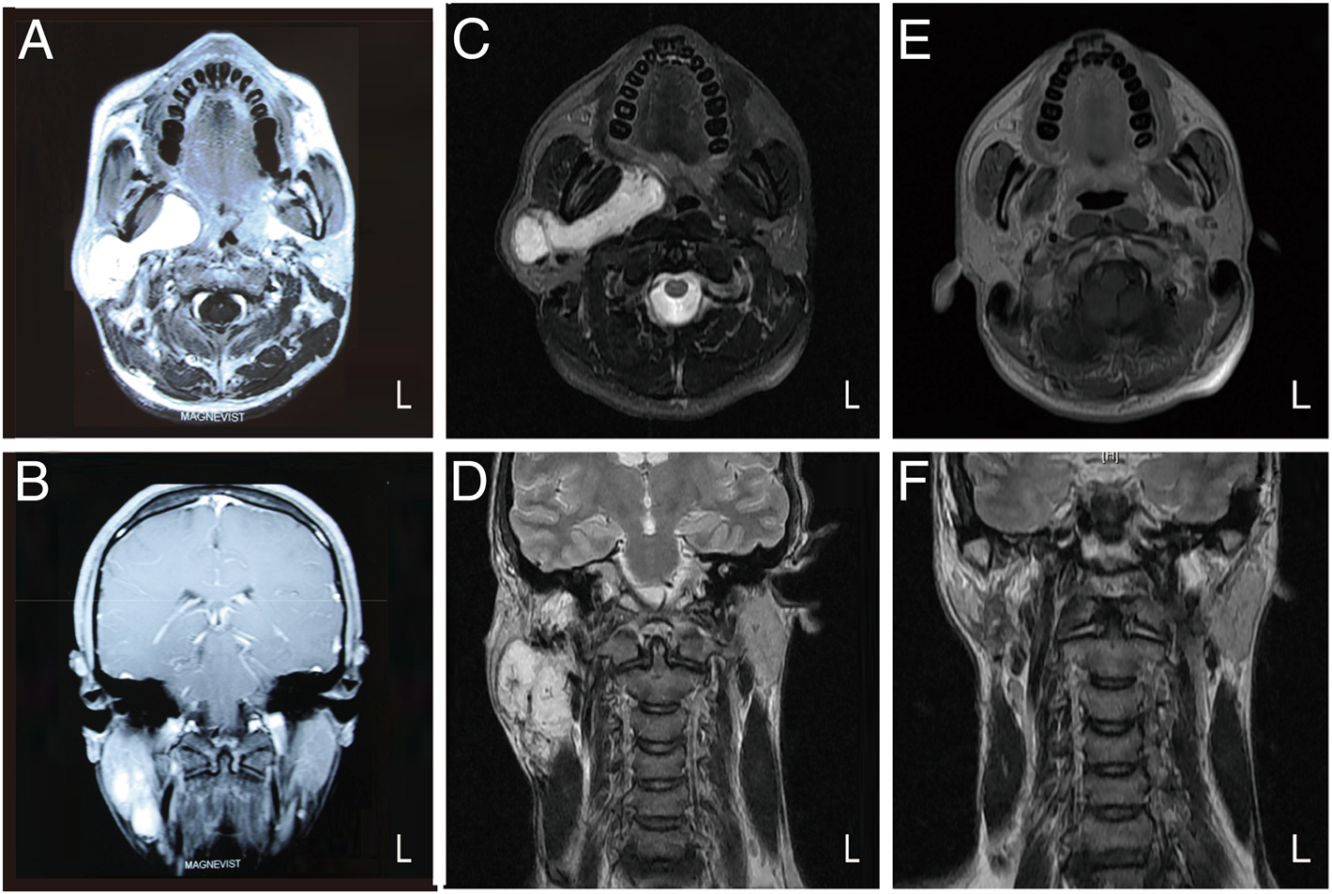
Giant cell angiofibroma. (A) Axial T1-weighted MRI of the tumor prior to sclerotherapy. (B) Coronal T1-weighted MRI of the tumor prior to sclerotherapy. (C) Preoperative axial T2-weighted MRI of the tumor after sclerotherapy. (D) Preoperative coronal T1-weighted MRI of the tumor after sclerotherapy. (E) Postoperative axial T1-weighted MRI at six months after resection showing no signs of recurrence. (F) Postoperative coronal T1-weighted MRI at six months after resection showing no signs of recurrence.
